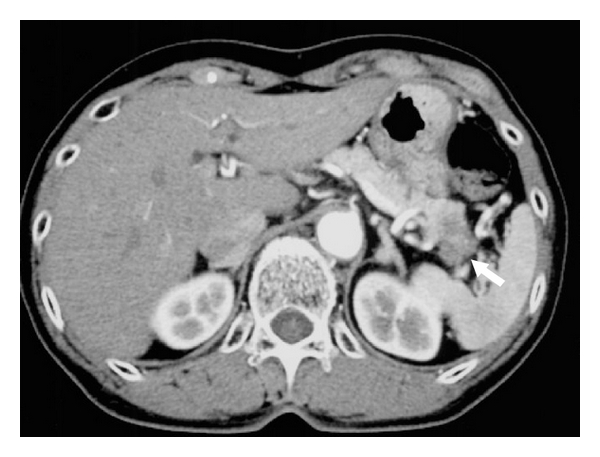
Case 2: Contrast CT 6 months after the first CT demonstrating a small tumor as a low-density area in the tail of the pancreas.
